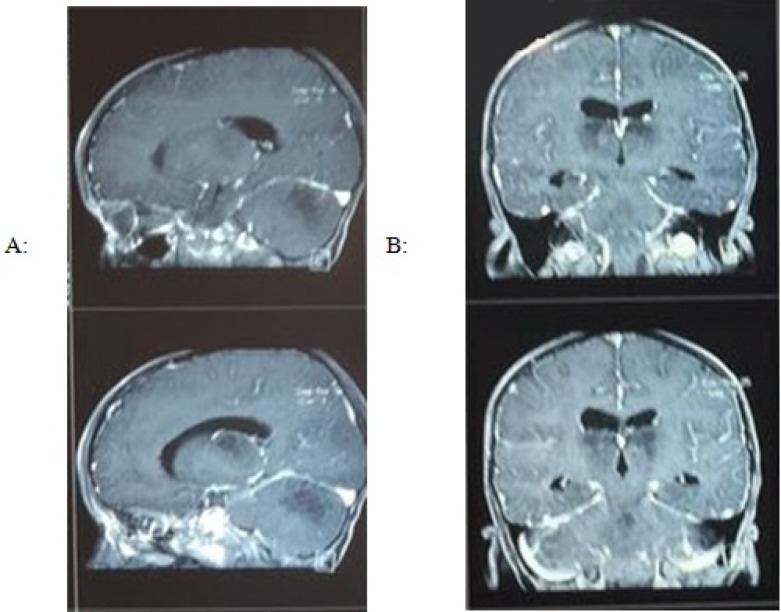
Sagittal (A) and coronal (B) views of brain MRI of thalamic lesion
